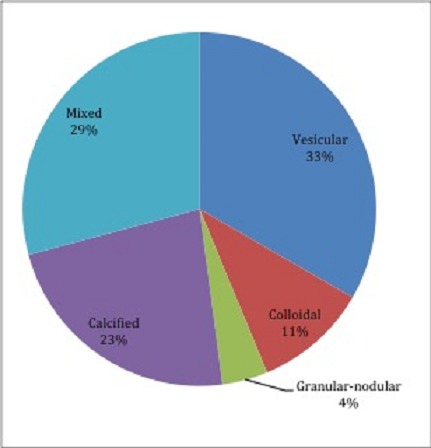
Shown is the distribution of neurocysticercosis lesions based on brain CT appearance and description. The most common brain lesions were vesicular cysts and the mixed type
